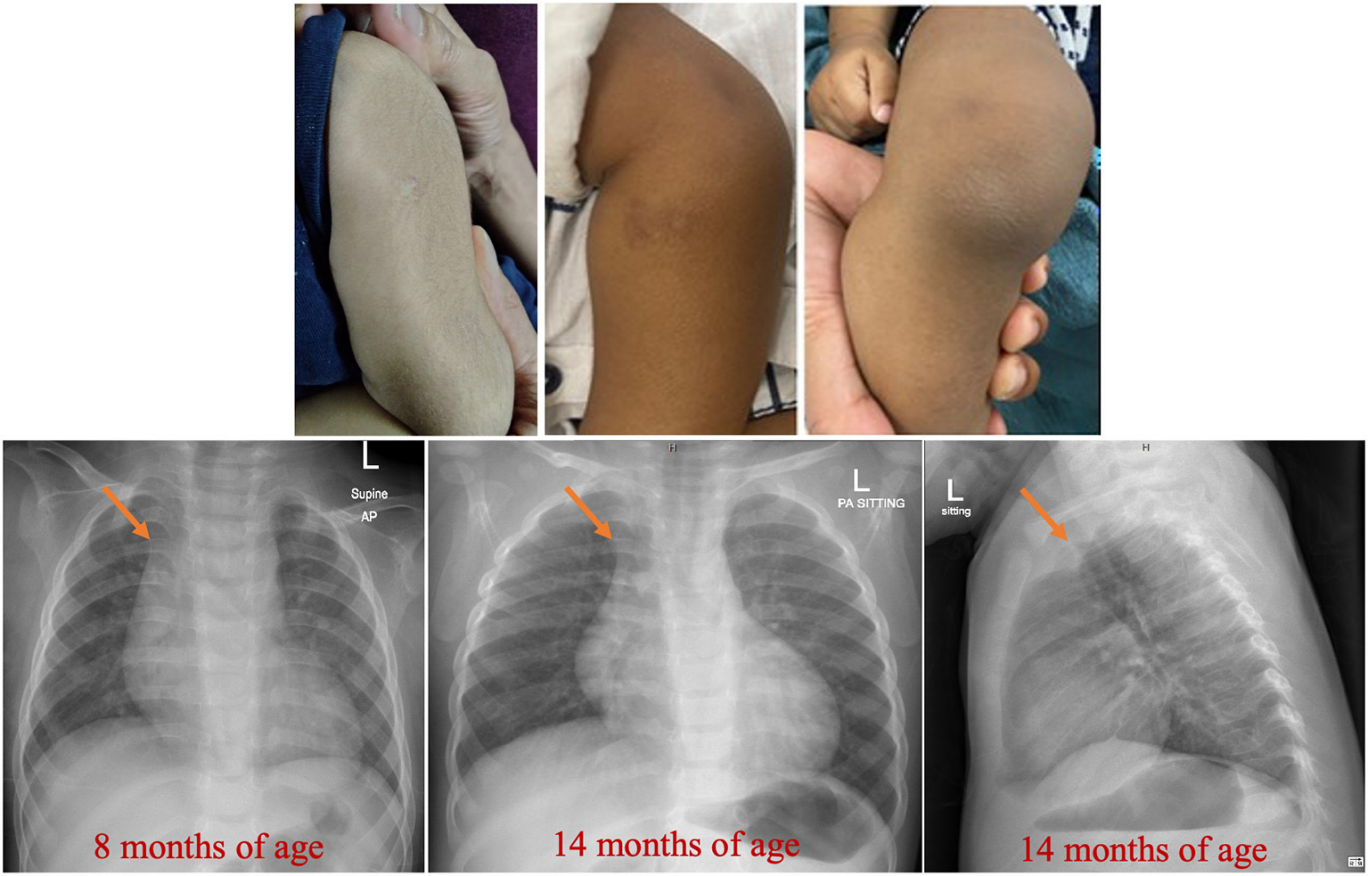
Findings on the skin and chest radiographs. Healed BCG site and dry skin with bruises and scars are evident. Prominent thymus (arrowed) at 8 mo of age is evident. Please also note the normal skeletal radiographs.
